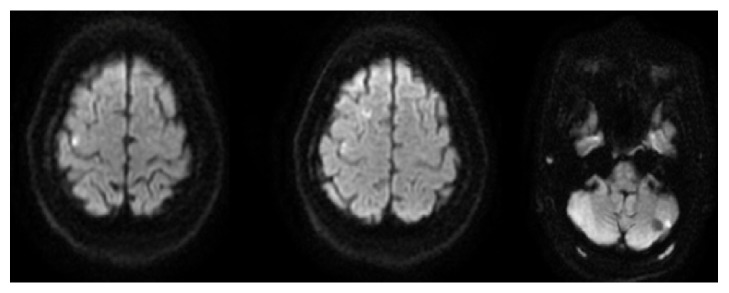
MRI of the brain without contrast showing small foci of diffusion restriction involving the right frontal lobe and left cerebellar hemisphere consistent with areas of acute infarctions.* Abbreviation.* MRI: magnetic resonance imaging.
